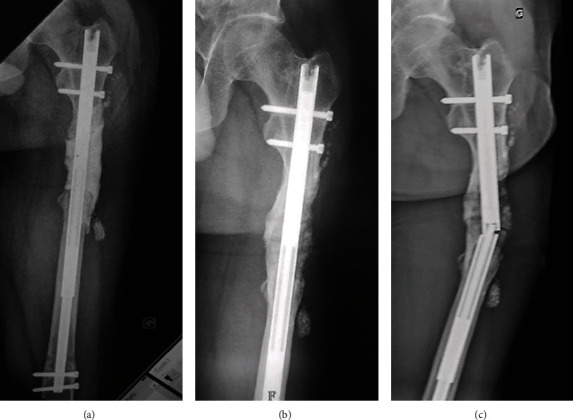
Second procedure. Radiographs showing the second cement spacer (a), the bone graft aspect at six months after the second grafting (b), and the subsequent elongation nail breakage that occurred four months later (c).
